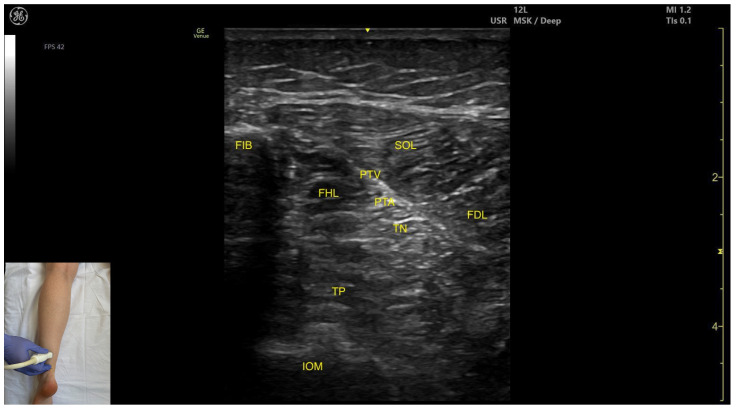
Ultrasound anatomy of the flexor hallucis longus: FIB—fibula; FHL—flexor hallucis longus; TP—tibialis posterior; IOM—interosseous membrane; PTA—posterior tibial artery; PTV—posterior tibial vein; TN—tibial nerve; SOL—soleus; FDL—flexor digitorum longus.
